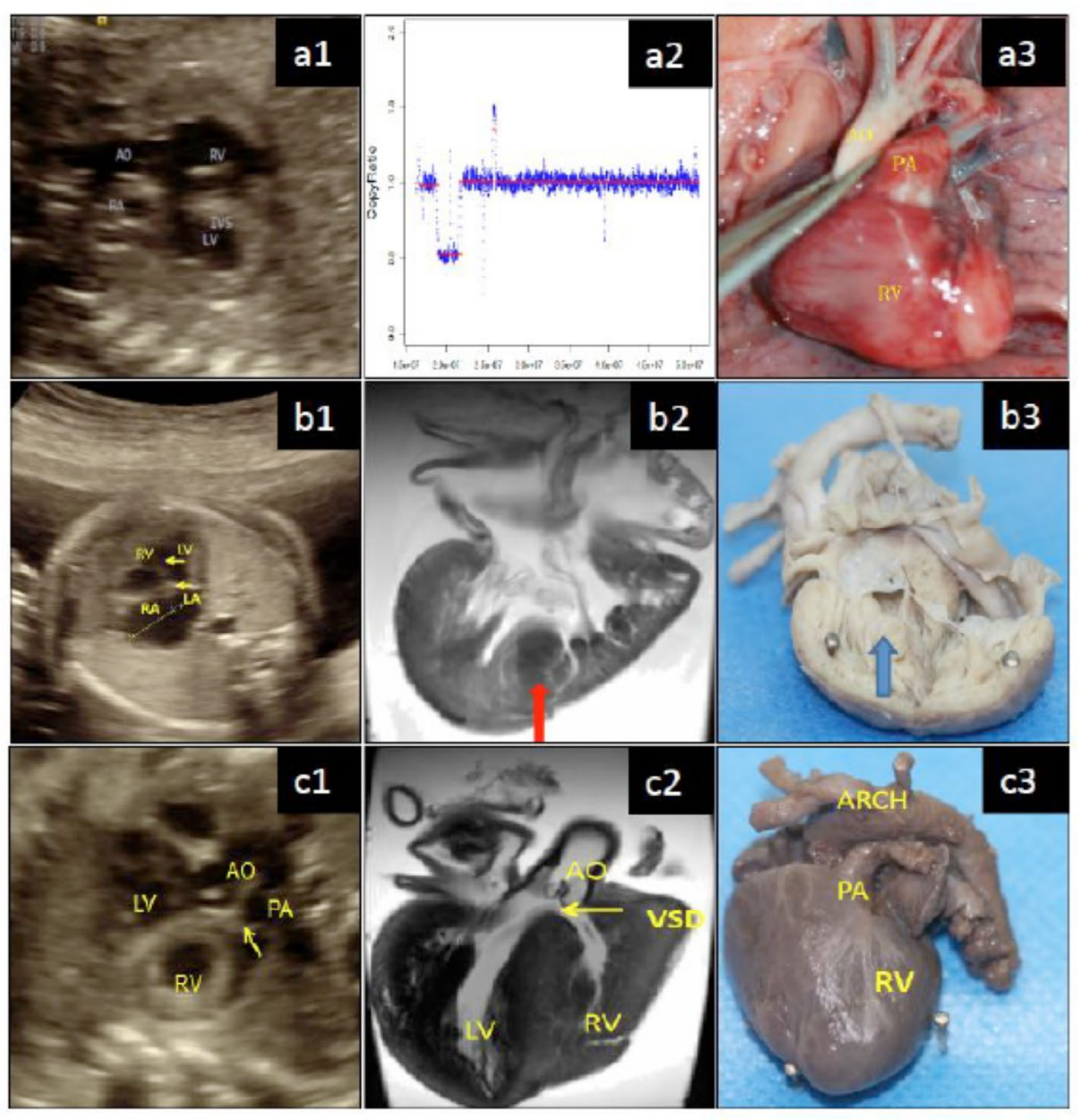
Comprehensive study of CHD. Prenatally identified CHD by fetal echocardiography (a1, b1, c1) was confirmed with MRI (b2, c2), pathological autopsy (a3, b3, c3), and genetic studies of microarray that showed microdeletion (a2) or genome wide sequencing (not shown). Three cases are presented to demonstrate the prenatally fetal-echocardiography-screening were verified and confirmed with MRI, autopsy, and genetic/genomic approach. Case 1 (a): DiGeorge syndrome was observed with DORV by prenatal ultrasound (a1) and verified in pathological autopsy (a3). Genetic study with a microarray (a2) determined a microdeletion of 2,825,77Kb at chromosome 22q11.21 between 22.18920346-21746118, which fell into the DiGeorge critical region (DGCR) that embeds 69 genes. Case 2 (b); CHARGE syndrome was observed with AVSD by prenatal ultrasound (b1) and verified in pathological autopsy (a3). Genetic study with a microarray (a2), confirmed by a 7T MRI (as pointed by arrow in b2), verified by a single nucleotide deletion c.6482del at gene CHD7 (NM_017780.3), which resulted in a frameshift mutation p.His2161Leufs54 and produced a mutant C-terminal peptide of CHD7 protein. Case 3 (c): Noonan syndrome (type X) was identified with a TOF presented by VSD, PA, and ARCH in the echocardiography (c1), which was confirmed by a 7T MRI (c2) and pathological autopsy (c3). GWS determined a single nucleotide mutation c.309C>A at gene LZTR1 (NM_006767.3), resulting in a nonsense mutation of p.Cyc103* that truncated C-terminus of LZTR1 protein
